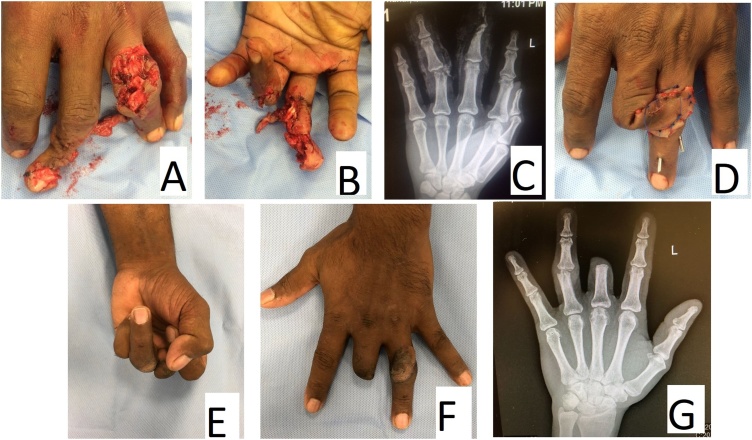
The first case A) and B) The severely crushed middle finger requiring amputation at the level of the proximal interphalangeal joint; and the soft tissue complex defect in the adjacent ring finger. C) X-ray showing the intraarticular fracture of the ring finger. D) Appearance after tendon repair, k-wire immobilization and spare-part fillet cross finger flap reconstruction. E), F), and G) Clinical radiological appearance at 6 months after injury.
